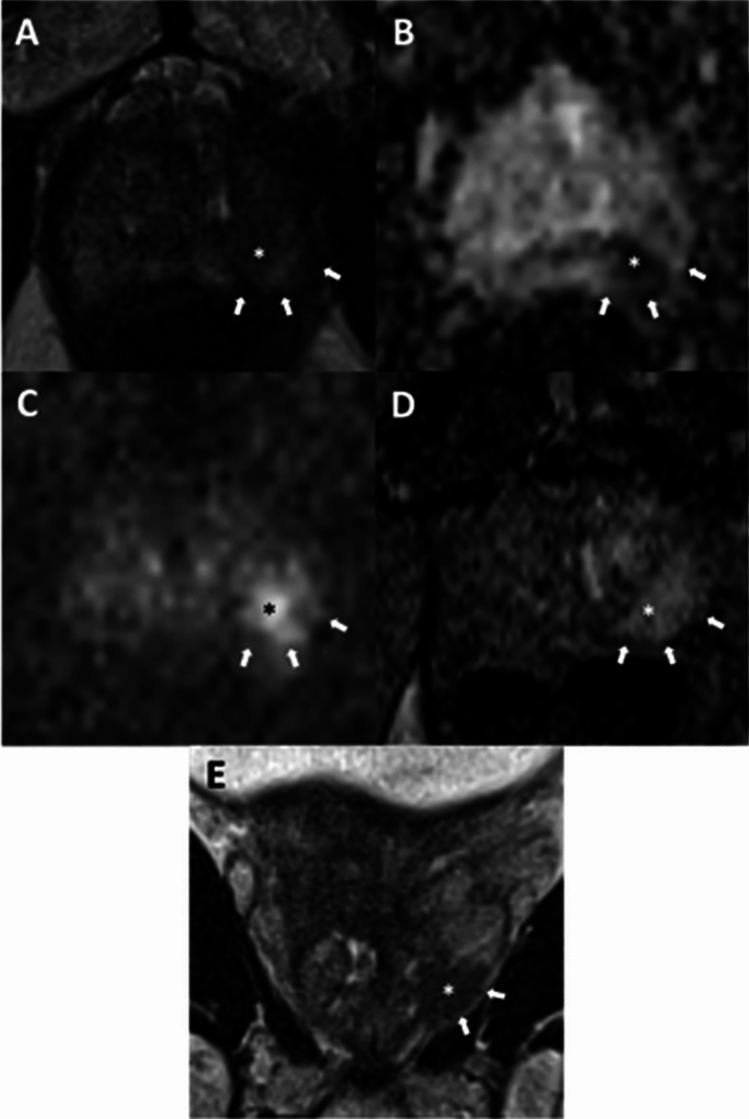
Multiparametric MRI of a 69-year-old patient with a serum prostate-specific antigen level of 8.2 ng/mL. T2WI had lower SNR and motion artifacts, whereas ADC map and high b-value DWI had lower SNR as well. AI classified T2WI as low-quality. The lesion (asterisk) was 1.7 cm in the left apical-base peripheral zone and assigned PI-RADS category 5. Long curvilinear contact length and capsular irregularity/bulge were noted (NCI EPE grade = 2) (arrows). Radical prostatectomy showed Gleason score 8 (4 + 4) prostate adenocarcinoma with no EPE, representing a false positive EPE call. Axial T2WI (A), ADC map (B), high b-value (b = 1500 s/mm^2^) DWI (C), dynamic contrast enhanced imaging (D), coronal T2WI (E). AI artificial intelligence, T2WI T2-weighted imaging, ADC apparent diffusion coefficient, DWI diffusion weighted imaging, PI-RADS Prostate Imaging Reporting and Data System, NCI National Cancer Institute, EPE extraprostatic extension, SNR = signal-to-noise ratio
